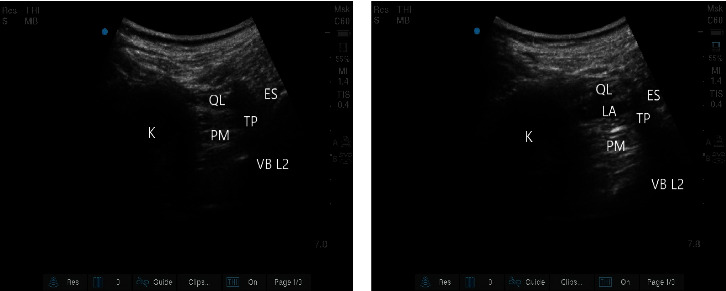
Ultrasonographic images of anterior QLB. (a) Ultrasound anatomical structure before block. (b) Spread of local anesthetic. K, kidney.
